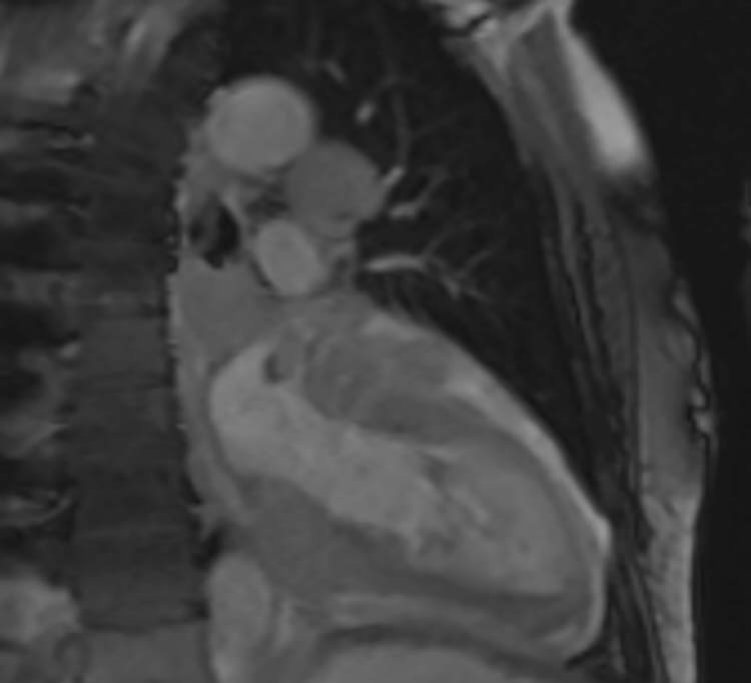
Cardiac MRI (sagittal plane) showing the lesion encasing the aorta, pulmonary artery and all coronary arteries of the heart.
